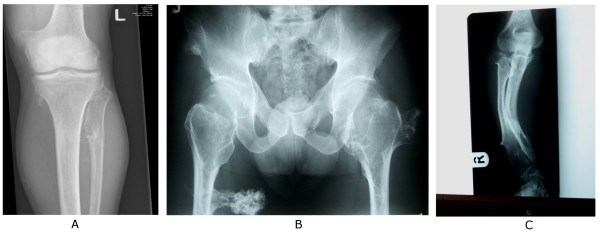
Examples of radiographs demonstrating multiple osteochondromas around the knee (A) and at the pelvis and proximal femur (B), while (C) demonstrates the deformity of the forearm (shortening of the ulna with secondary bowing of radius) that is found in 39–60% of the patients.
